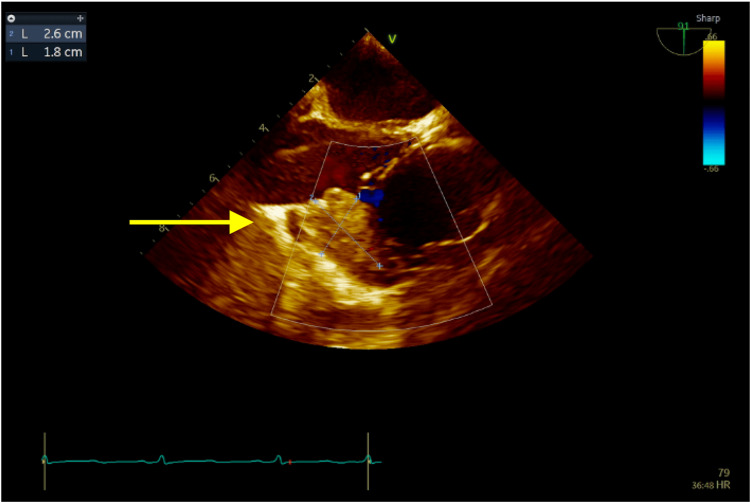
Transesophageal echocardiogram (TEE) image The image shows an irregularly shaped right atrial 2.6 cm x 2.3 cm x 1.0 cm (anterior-posterior, transverse, craniocaudal) peripherally hypointense lesion with a gray etched core close to the crista terminalis. On phase-sensitive inversion recovery sequence, the entire lesion demonstrates homogeneous low signal compatible with thrombus. There is no lesional contrast enhancement.
